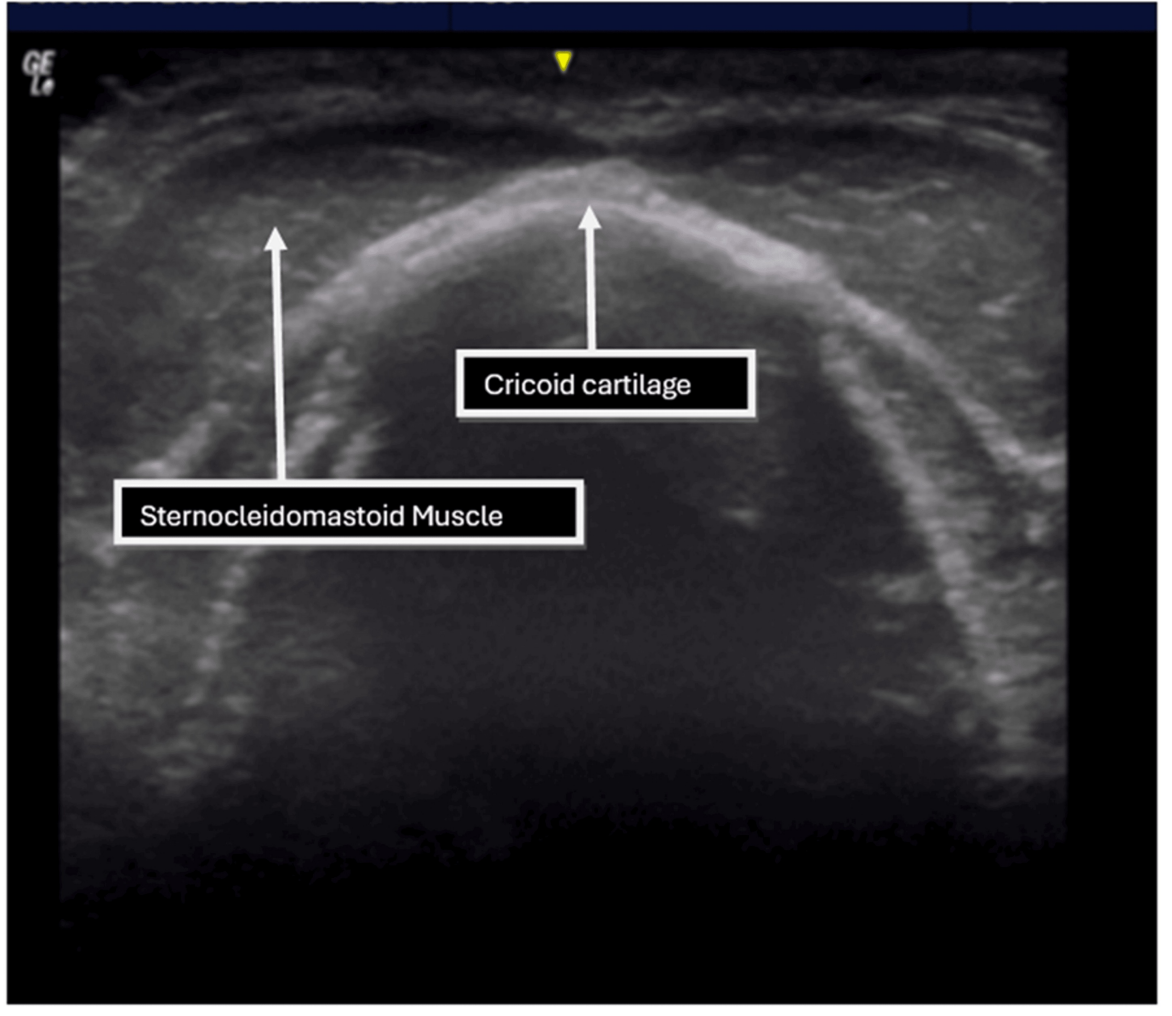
Transverse ultrasound image at the level of the superior border of the cricoid cartilage.
